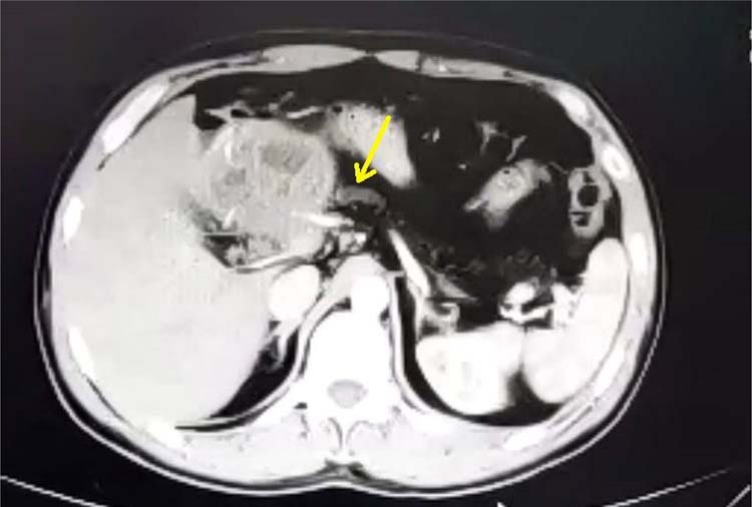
Axial contrast-enhanced CT scan of the abdomen showing dilatation of the MPD (5 mm).
